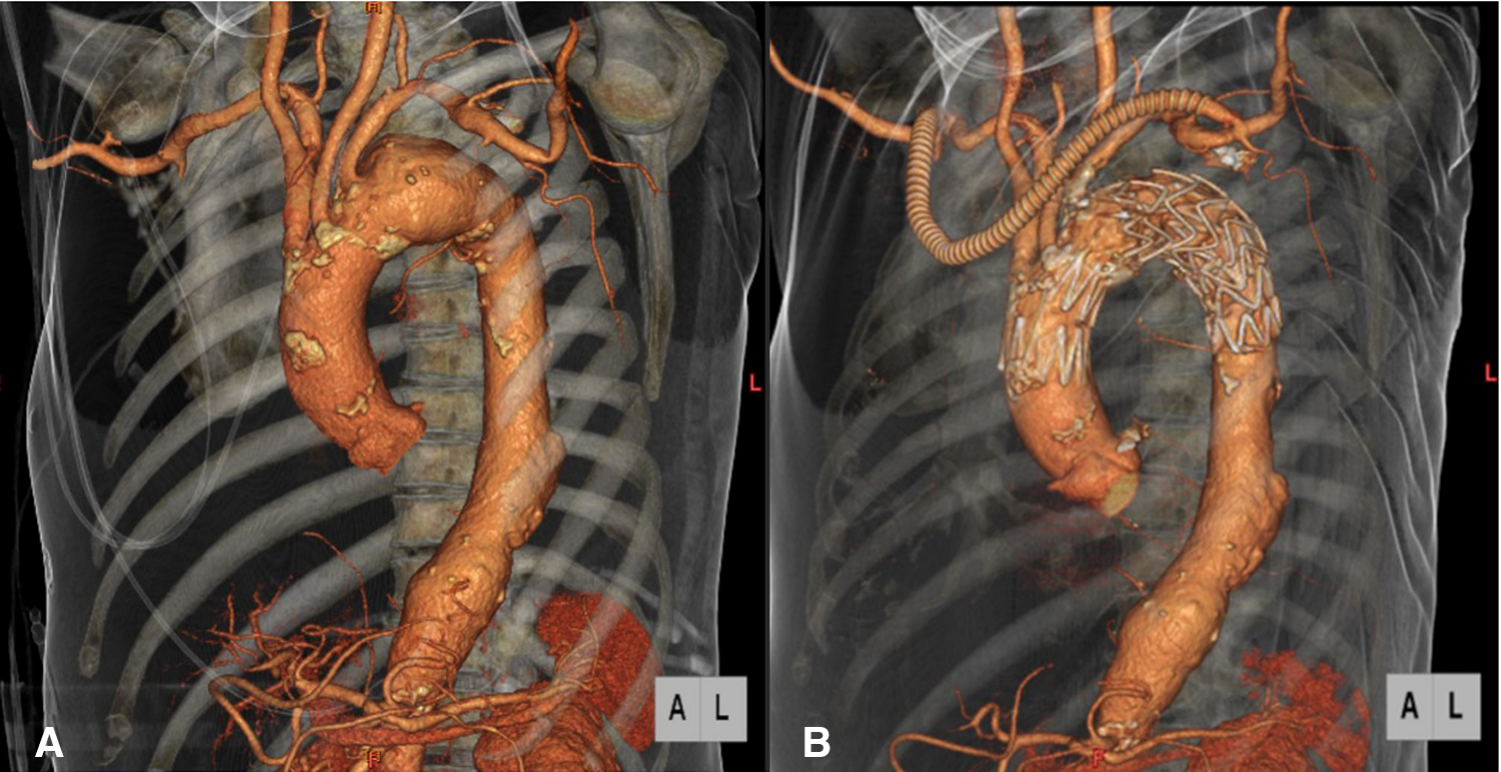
Preoperative CT angiography showed a distal arch aneurysm which involved the left subclavian artery (A). Postoperative CT angiography showed patent debranching bypass and exclusion of the aneurysm with the Najuta thoracic stent graft system and Valiant Captivia, with no bird-beak phenomenon in the ascending aorta (B).
